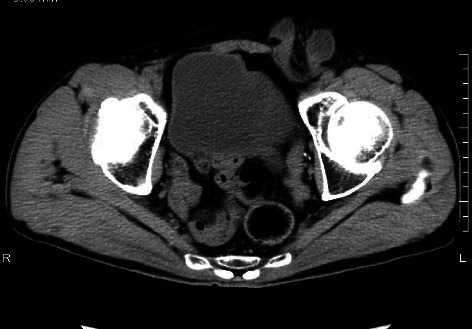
CT findings at the time of presentation (Case 1: intestinal incarceration in the left inguinal region).
